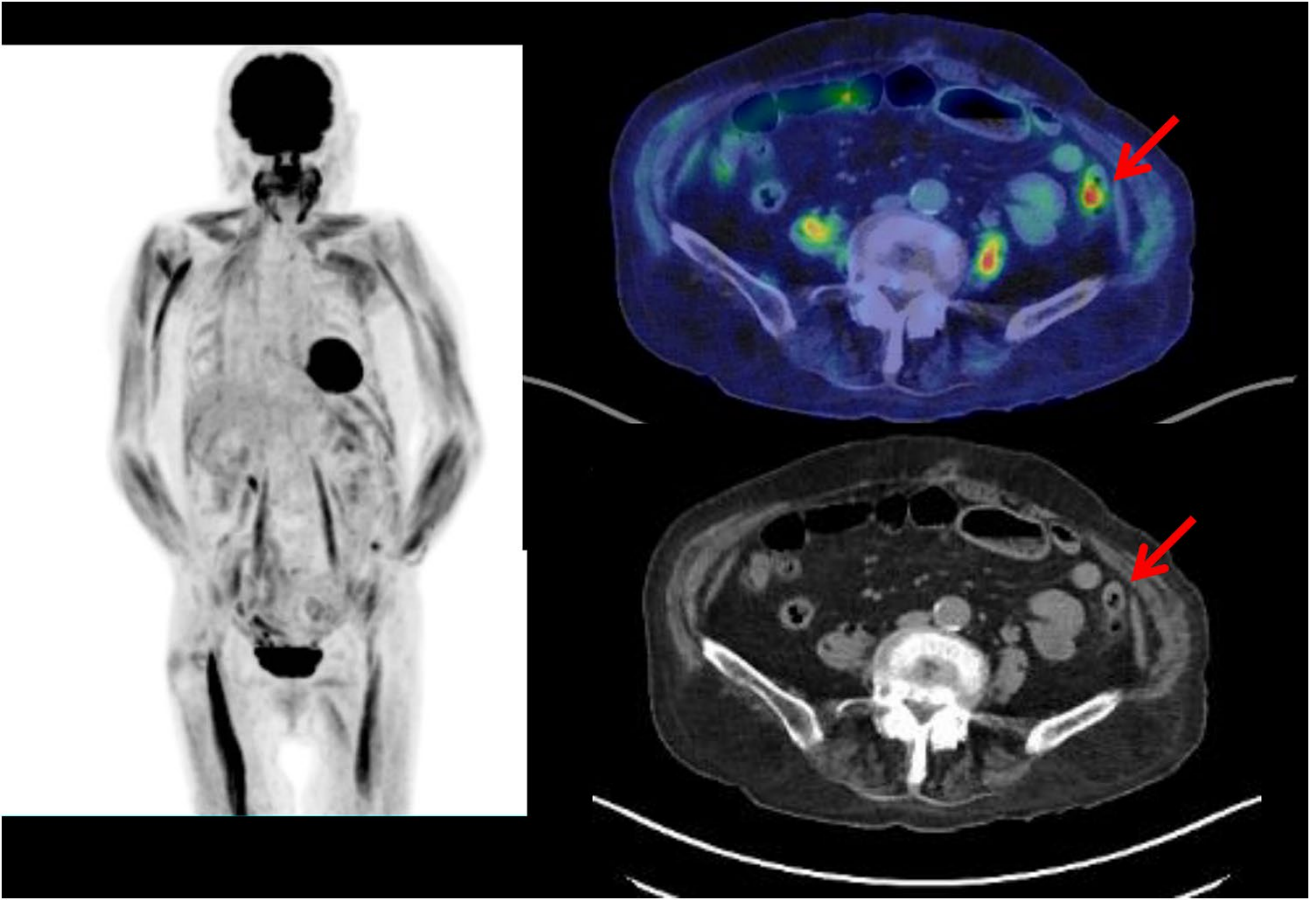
A 71-year-old female with transcriptional intermediary factor 1-positive dermatomyositis was treated with corticosteroid. This patient had a history of thyroidectomy for thyroid cancer 7 years ago. Whole-body FDG PET maximum projection image showed increased FDG uptake in systemic muscles. Cross-sectional FDG PET/CT images showed focal FDG uptake (SUVmax 5.7) in the descending colon, which was diagnosed as colon cancer by endoscopic biopsy (arrows)
